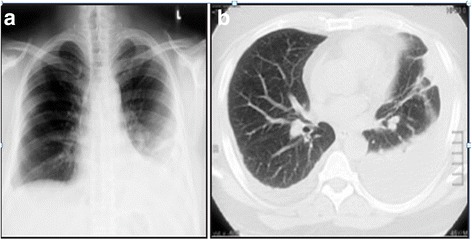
a Chest X-ray and b computed tomography scan of the chest showing bilateral pleural effusions, more significant on the left side
